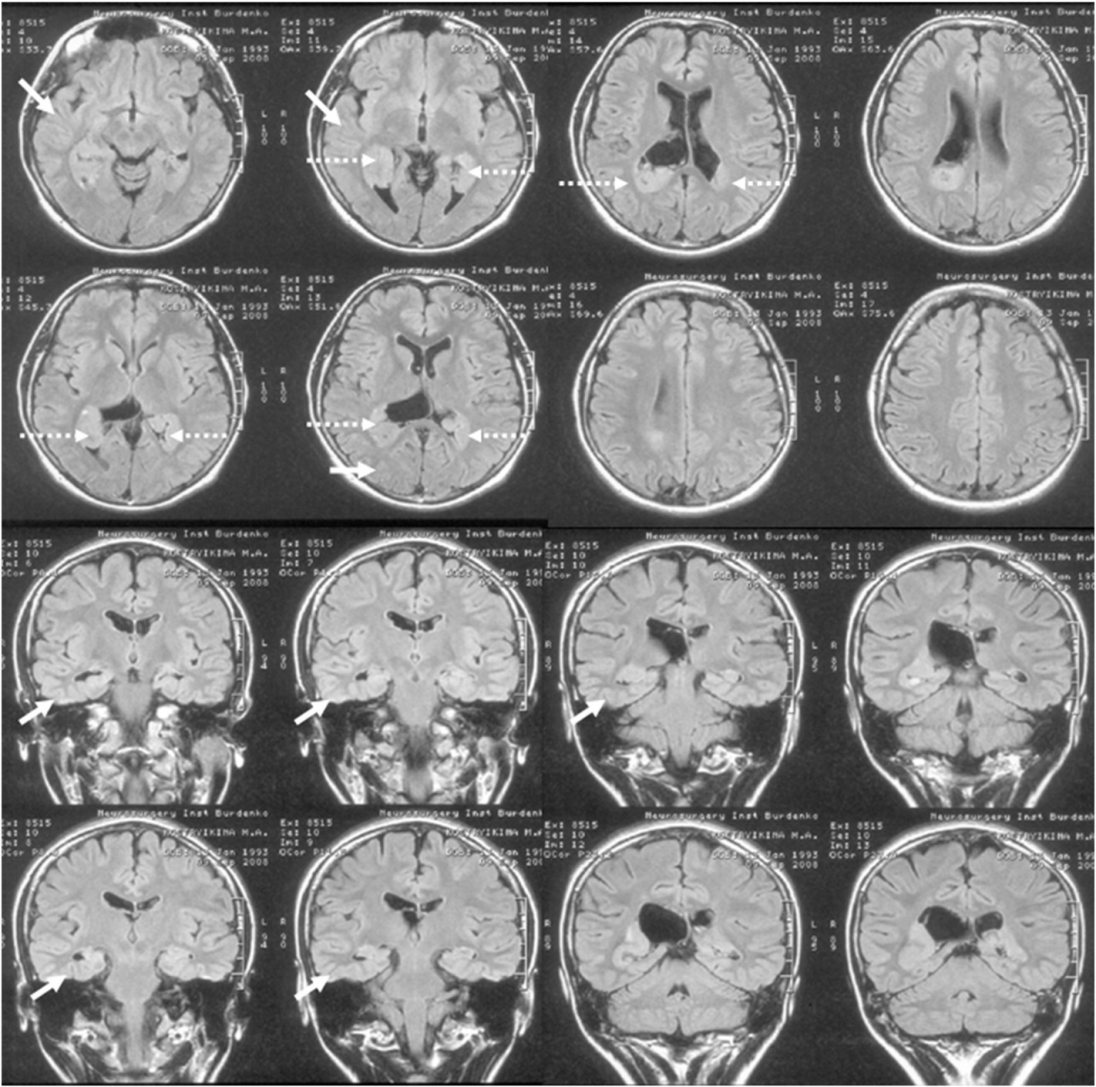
Magnetic resonance imaging findings in the patient: regions of cortical dysplasia (arrows); nodules of heterotopic matter (interrupted arrows).
